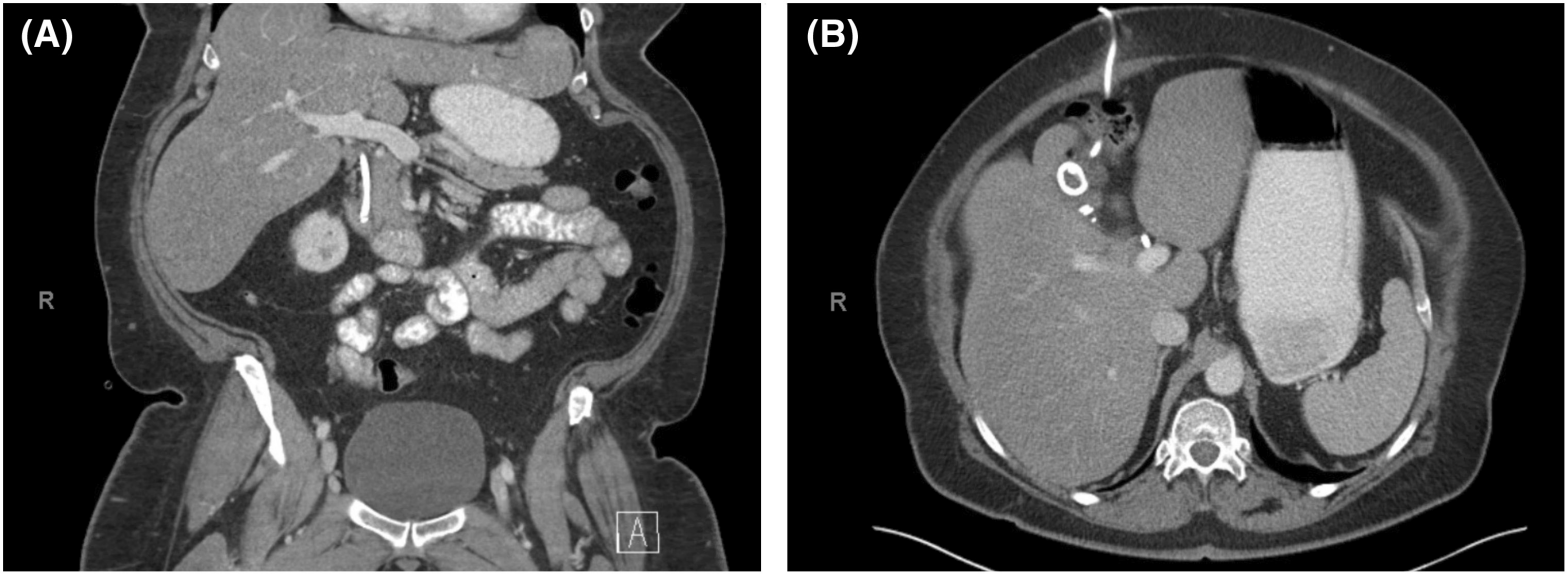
Preoperative abdominal CT demonstrating biliary stent in common bile duct (A). A right upper quadrant abdominal drainage catheter is in place and terminates in the gallbladder fossa (B)
